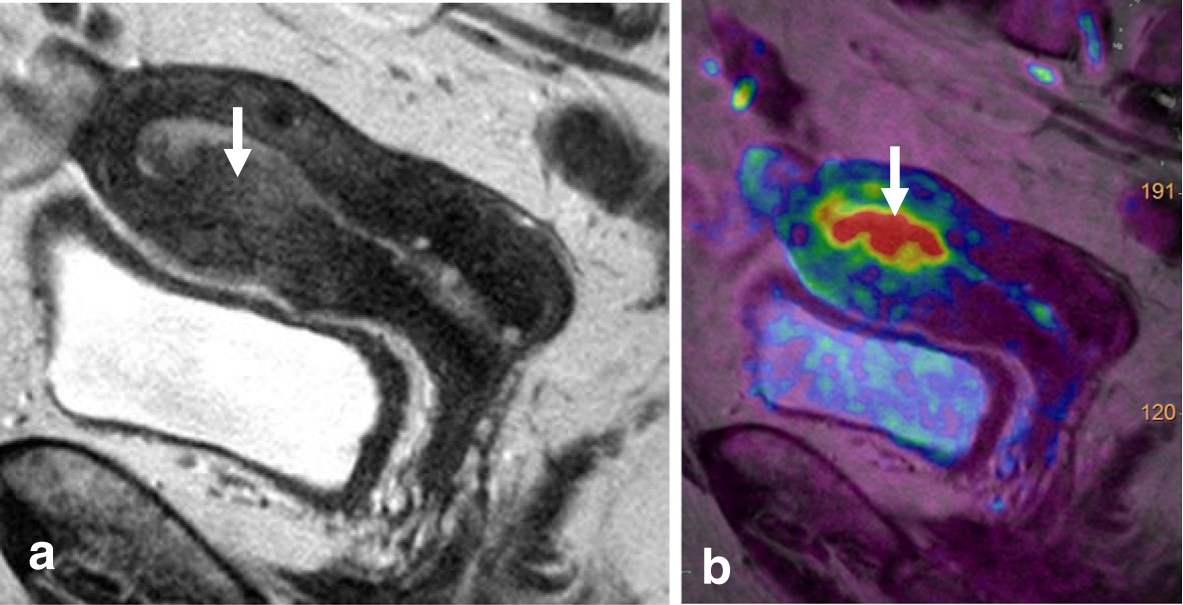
FIGO IA endometrial tumor (arrows) with myometrial invasion of < 50% is isointense in T2WI images (a) and well-delineated in fused T2WI-DWI images (b)
